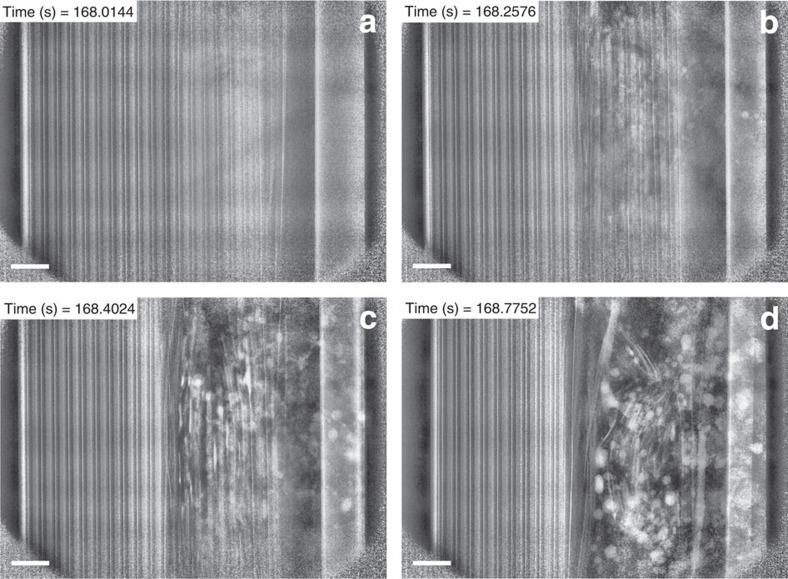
Radiographs from Supplementary Movie 5 showing the propagation of thermal runaway in Cell 1. (a) Radiograph of the *YZ* plane before thermal runaway; (b,c,d) sequential images showing the propagation of thermal runaway through the cell. The thermal runaway initiates at the inner layers where the maximum temperature is apparent and spreads radially outwards. The formation of copper globules can be observed as highly attenuating white blots in images b, c and d. Heating is applied from the right of the images but continuous rotation at 180° every 0.4 s maintains an even circumferential temperature distribution. Scale bar, 1 mm.
